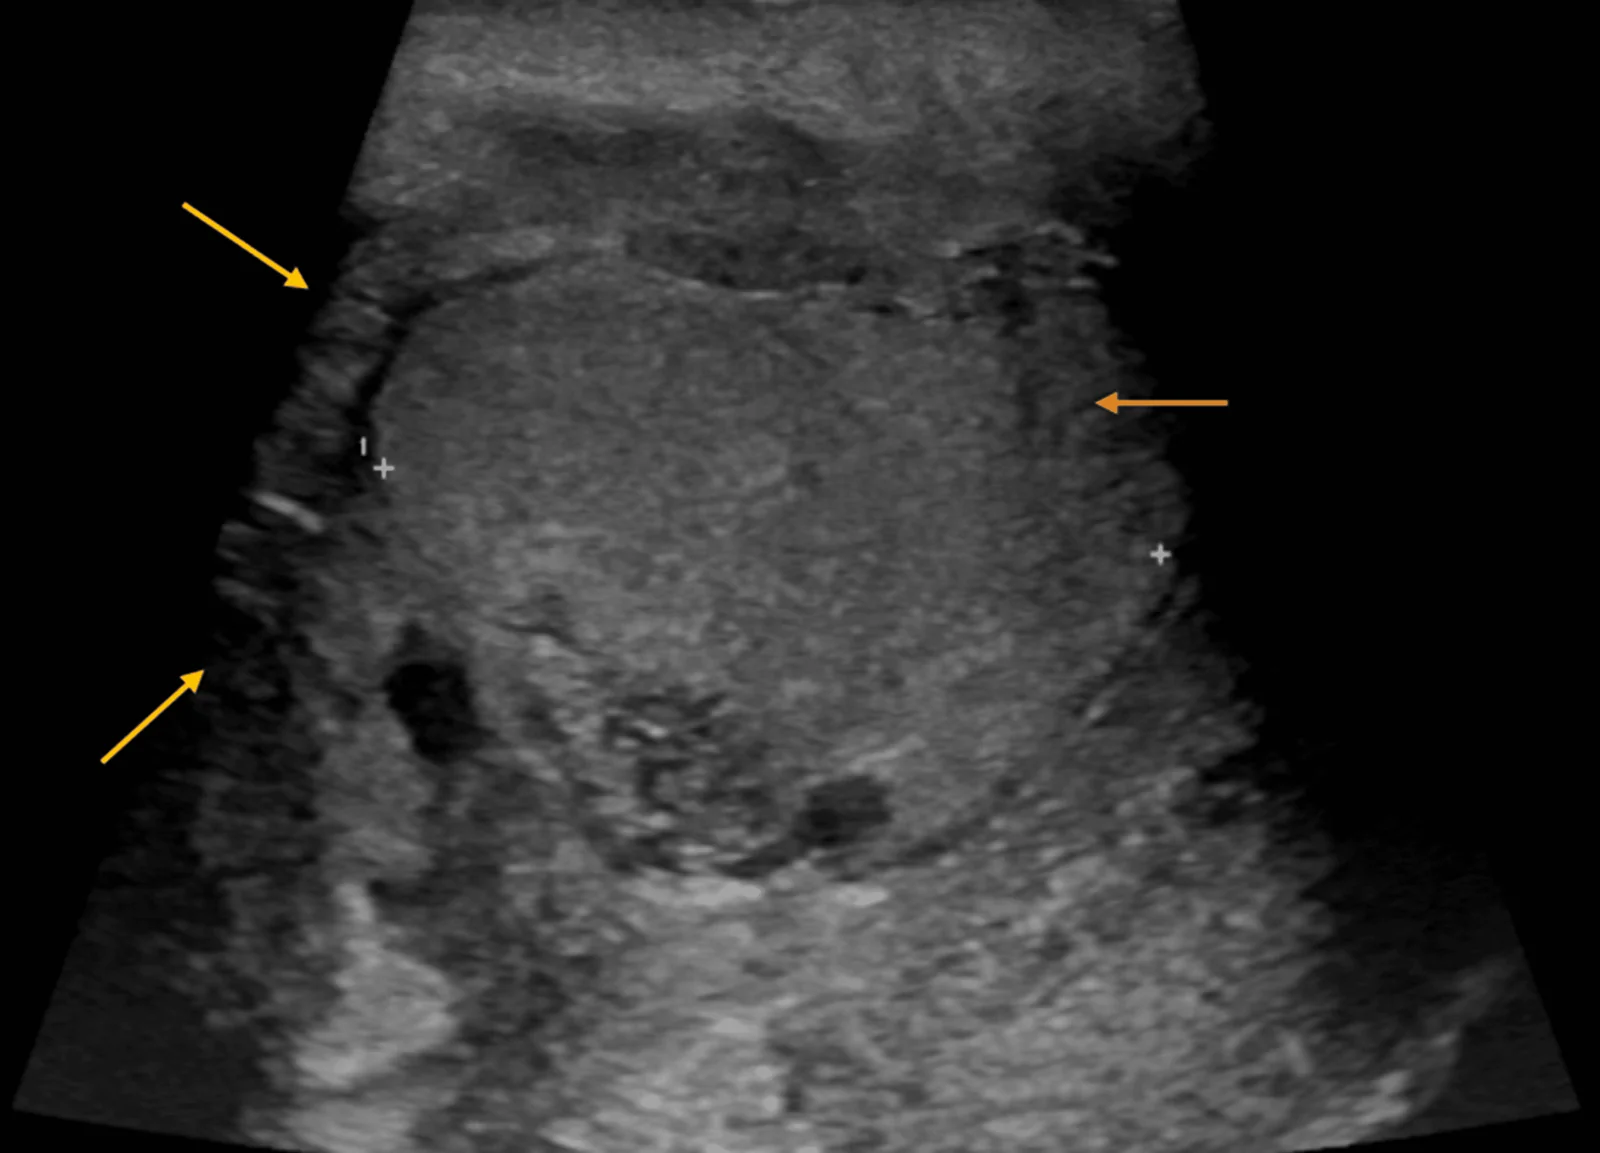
Ultrasound image of the right testis (orange arrow) showing multiple polypoid nodules arising from the tunica vaginalis (yellow arrows).
